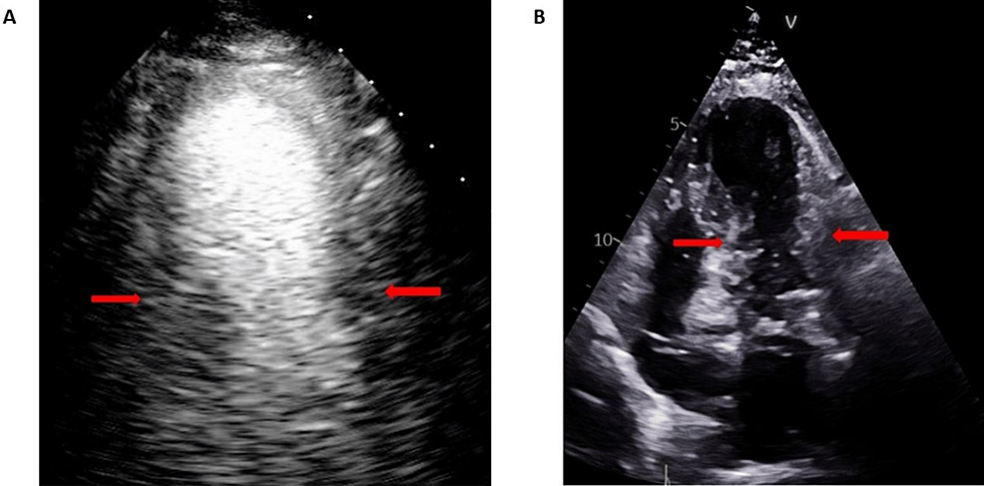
Patient's TTE A: TTE with contrast showing ballooning of left ventricle apex due to severe hypokinesis, left ventricle mid to apical segments with relative hyperkinesis of the base; B) TTE without contrast demonstrated no left ventricle thrombus TTE: Transthoracic echocardiography
